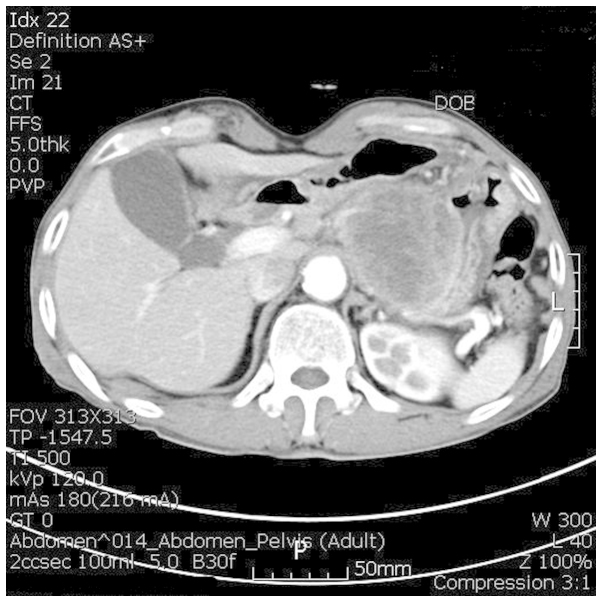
Computed tomography revealing a heterogeneously-enhanced lesion of the cardia.
